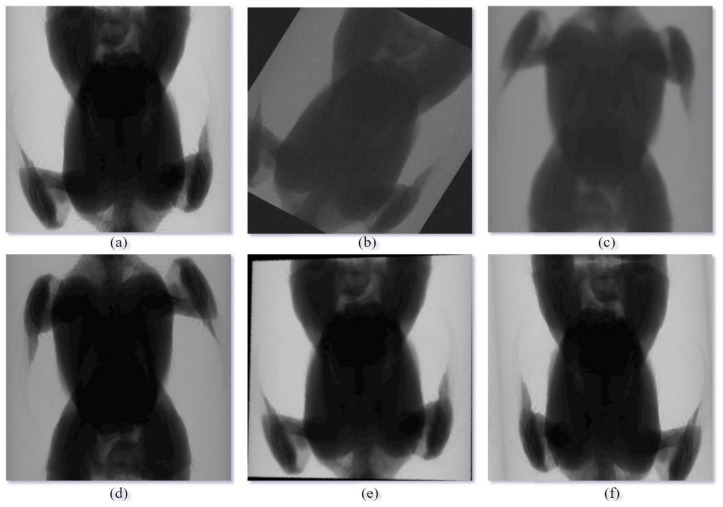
X-ray image augmented example. (a) Original; (b) Random rotation; (c) Vertical flip; (d) Horizontal flip after vertical flip; (e) Perspective transform; (f) Elastic transform after horizontal flip.
